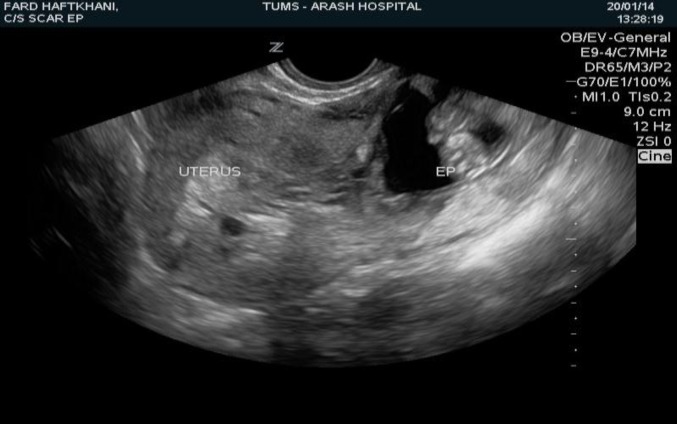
Transvaginal ultrasonography showing an empty uterine cavity with the gestational sac containing a fetus alive in the lower segment of the uterus (Cesarean scar pregnancy)
